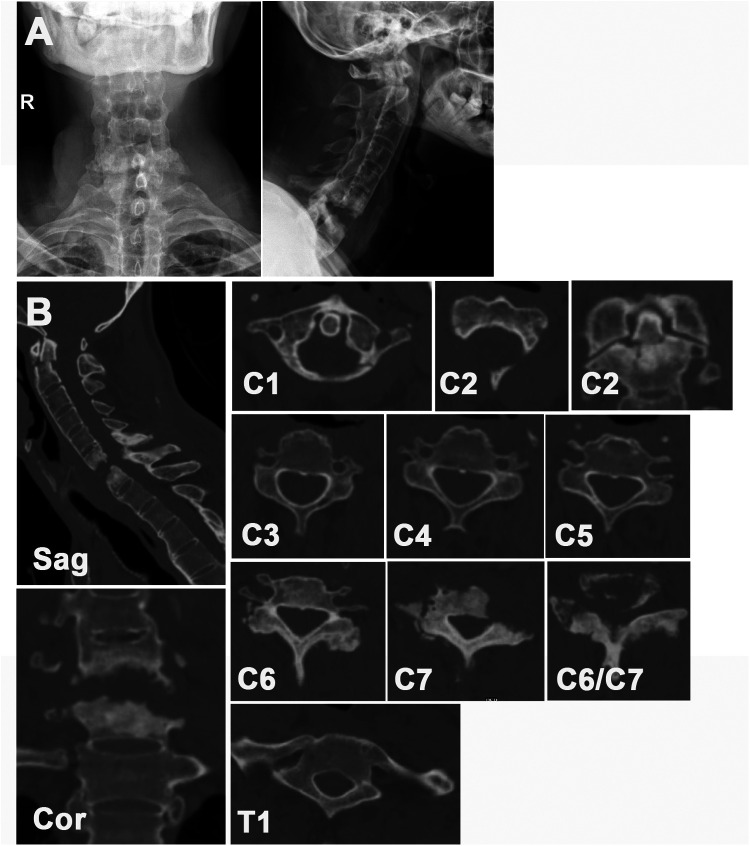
(A) preoperative cervical x-ray image. (B) Preoperative cervical CT image.
